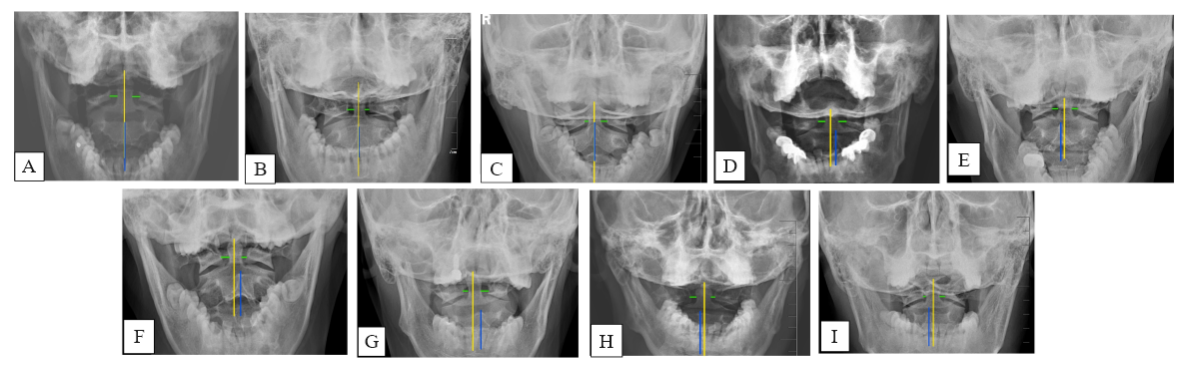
Comprehensive classification of atlantoaxial joint subluxation using X-ray. The green line indicates the distance between the lateral mass of the atlantoaxial joint and the odontoid process. The blue line indicates the axis line of the C2 spinous process. The yellow line indicates the midline of the spinous process. (A) Normal atlantoaxial joint; (B) Type A; (C) Type B; (D) Type C; (E) Type D; (F) Type AC; (G) Type AD; (H) Type BC; (I) Type BD.
